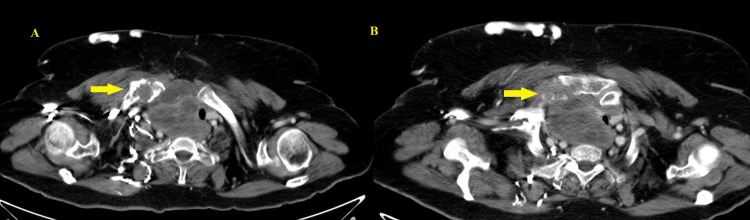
CT imaging from May 2025 A large predominantly cystic lesion was identified in the right lower neck with the involvement of the adjacent right thyroid gland as well. This shows heterogeneous osseous expansion with the involvement of the medial end of the clavicle (A) and right sternoclavicular joint with its erosion (B). CT: computed tomography
